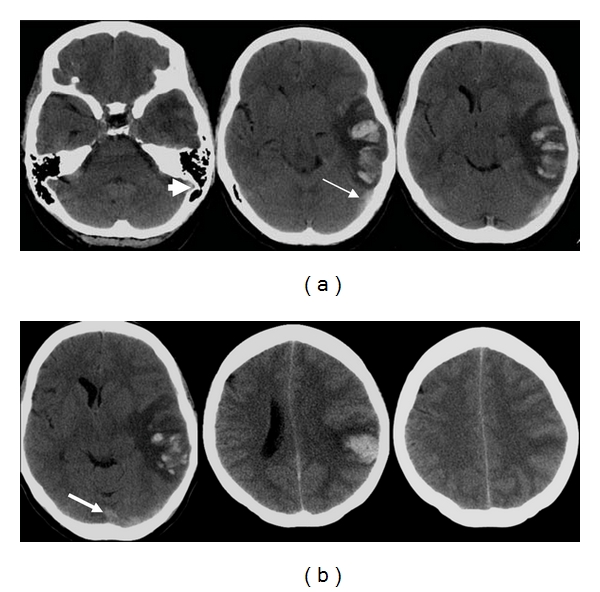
57-year-old woman presented with dysphasia, headache, and vomiting. Axial unenhanced CT images show hemorrhage in the left temporal parietal area. Apart from the typical temporal location suggesting this may be a venous hemorrhage, there is also high attenuation in the left sigmoid sinus (arrowhead), left transverse sinus (thin arrow), and straight sinus (thick arrow). MR venogram confirms occlusion of these sinuses (not shown).
